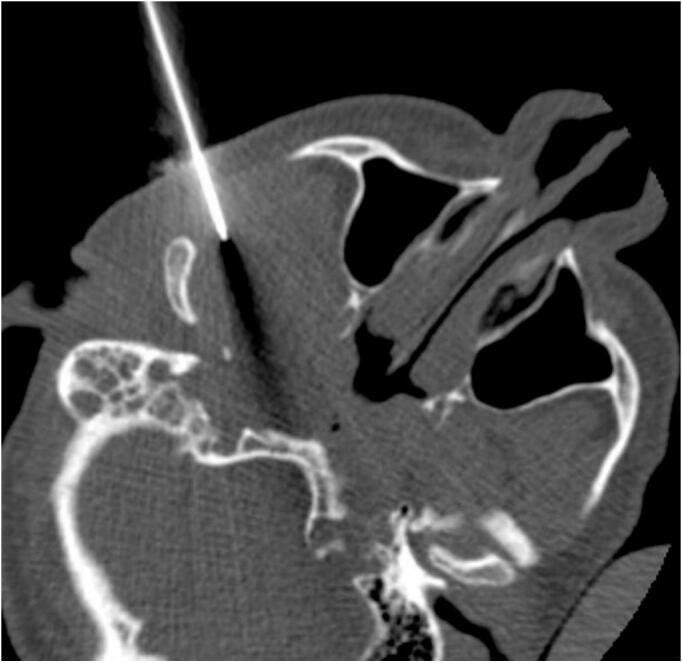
CT-guided percutaneous true-cut biopsy of the right ITF mass.
